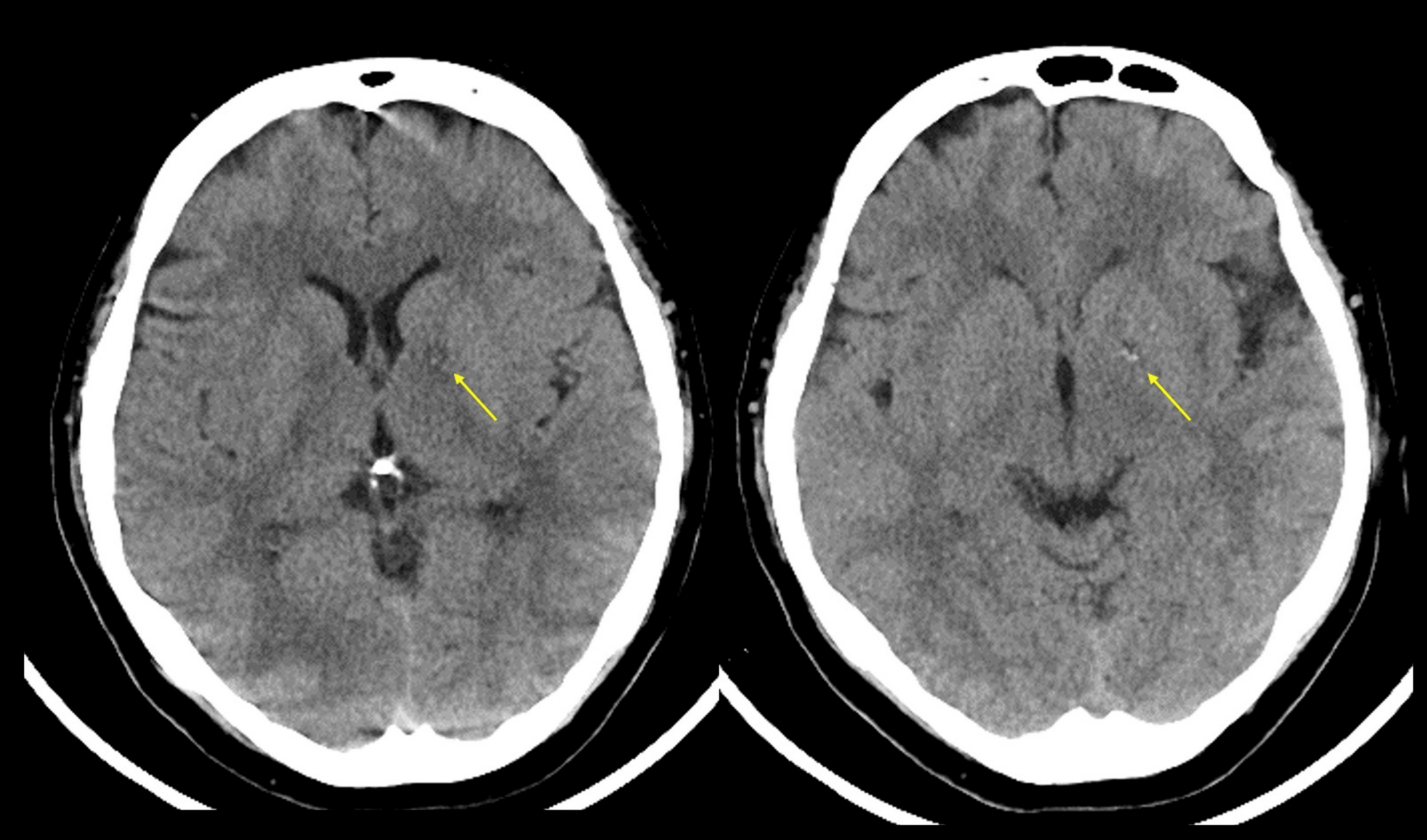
The result of brain CT. Brain CT on initial onset showing a low-density area mixed with a high-density area in the left globus pallidus. CT, computed tomography
